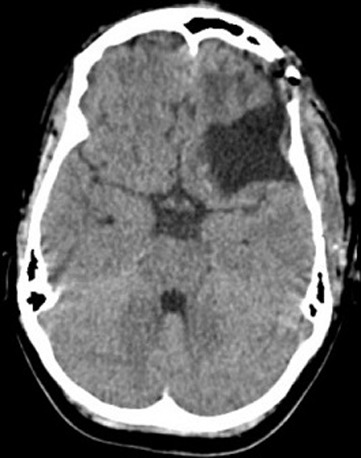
axial view of CT brain showing post-operative changes
